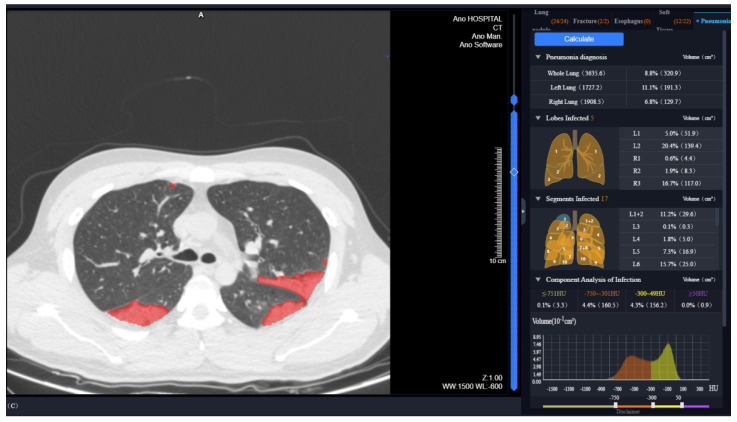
Quantitative analysis of CT images at discharge in uAI intelligent assistant system.
